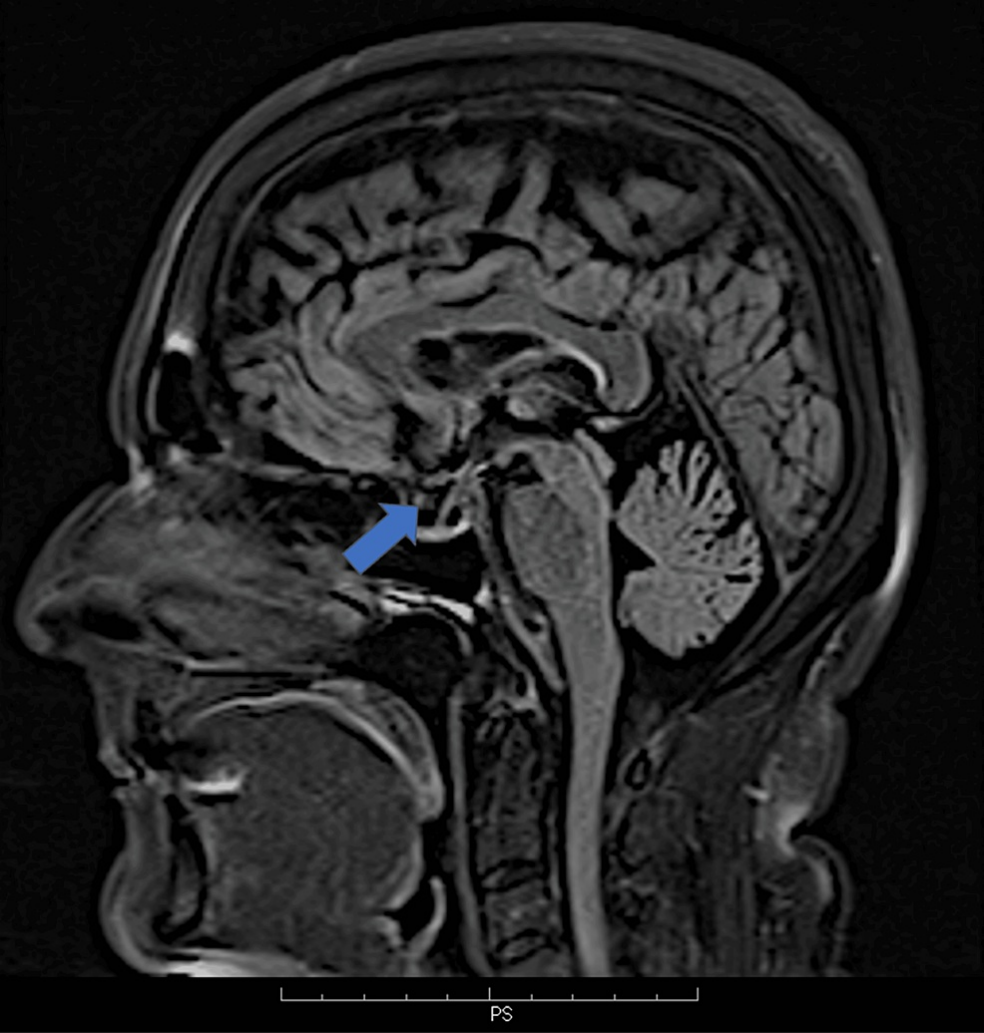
Sagittal FLAIR images of a 63-year-old patient with TGA and bilateral PAC The blue arrows show the compressed pituitary gland and its pituitary stalk
